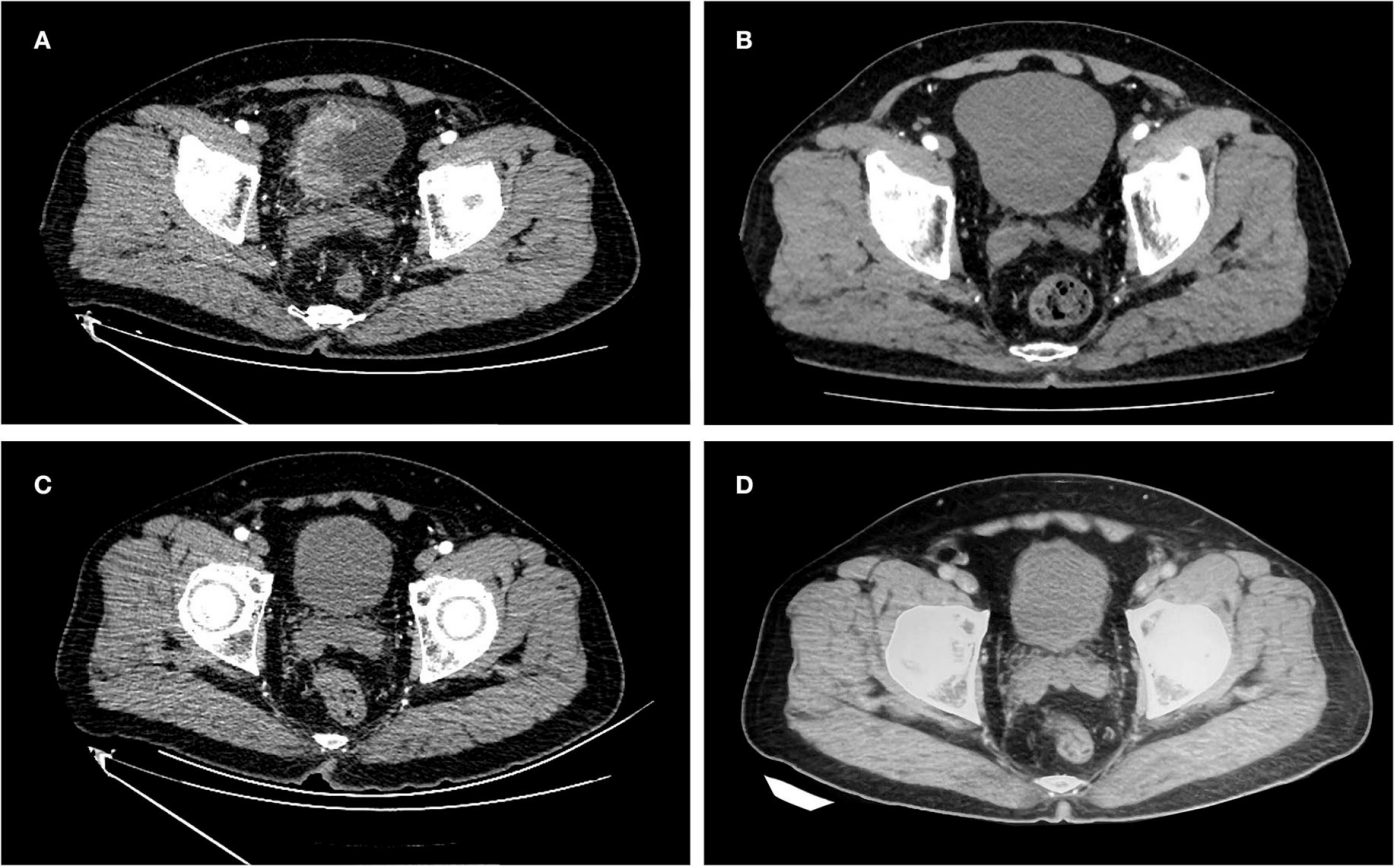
Images of the case 1 patient throughout the treatment. (A) Before treatment, abdomen enhanced CT showed localized invasion of the right wall lump of the bladder into the surrounding adipose tissue, invasion of the anterior bladder wall, and interruption of the continuity of the muscle layer. (B) After two courses of neoadjuvant chemotherapy, abdomen enhanced CT revealed a significantly reduced lump in the right wall of the bladder. (C) After maximal TURBT combined with tislelizumab, no tumor was seen on abdomen enhanced CT. (D) On February 18, 2022, the last follow-up abdomen enhanced CT showed no tumor recurrence.
